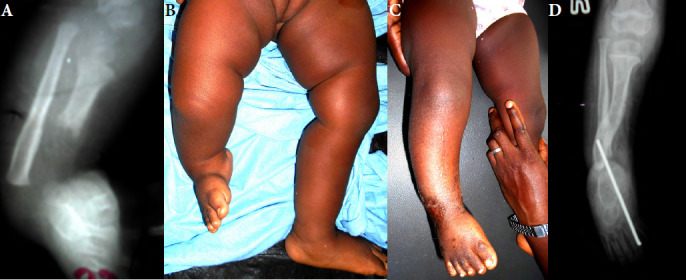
Aplasie partielle du tibia droit. L’enfant est vue à 12 mois; une tibialisation basse est réalisée à 15 mois, suivie d’une tibialisation haute à 24 mois. (A, B) Aspect clinique et radiographique pré-opératoire. (C, D) Aspect après tibialisation haute et basse
